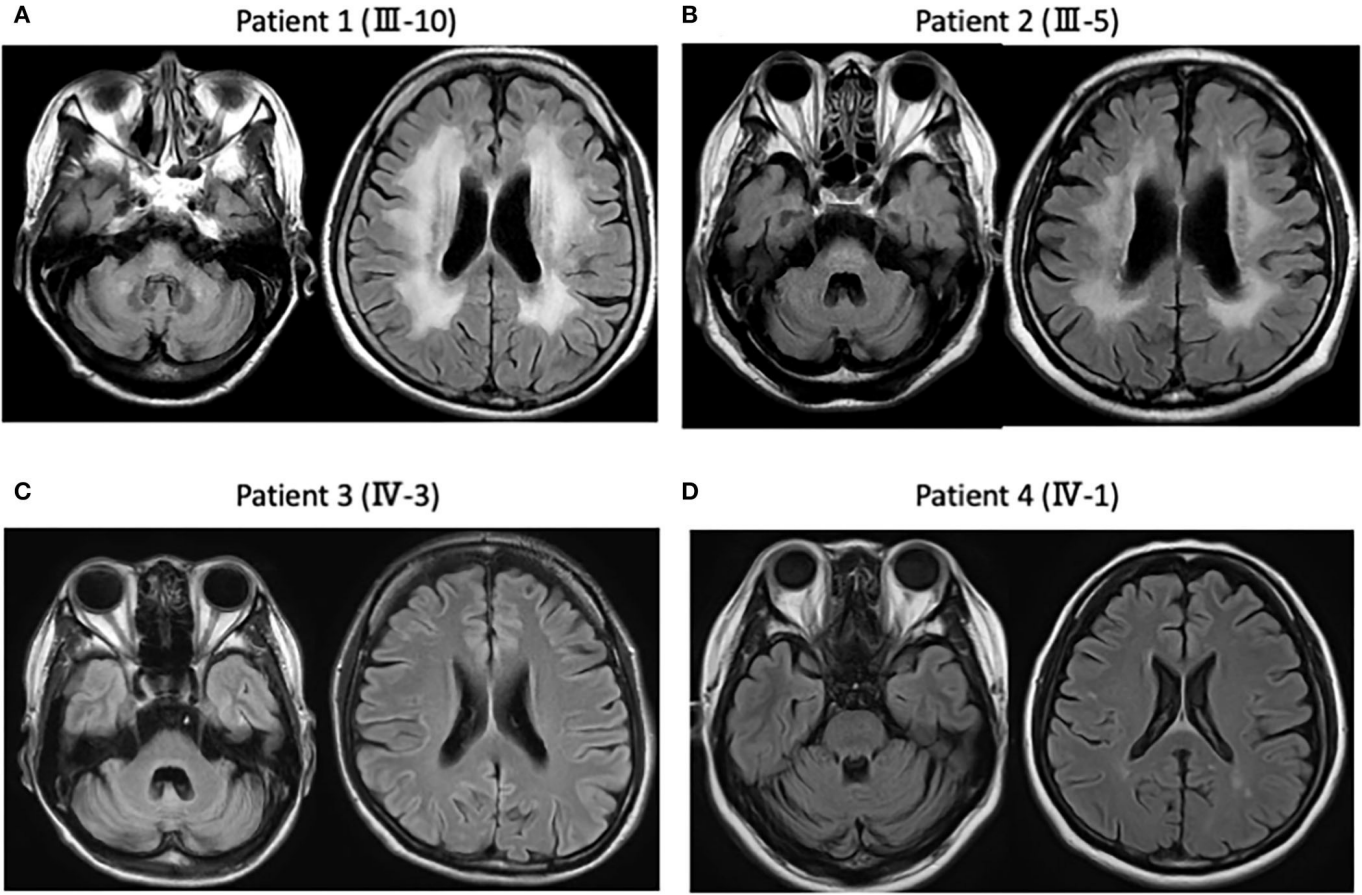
Magnetic resonance imaging (MRI) findings in the present four patients. (A) Patient 1 (III-10). Fluid attenuated inversion recovery images of the MRI show diffuse cerebellar and brainstem atrophy, as well as high-intensity signals in the diffuse periventricular white matter. (B) Patient 2 (III-5). MRI shows diffuse cerebellar and brainstem atrophy, as well as high-intensity signals in the diffuse periventricular white matter. (C) Patient 3 (IV-3). MRI shows diffuse cerebellar and brainstem atrophy without white matter changes. (D) Patient 4 (IV-1). MRI shows diffuse cerebellar atrophy without periventricular white matter changes.
